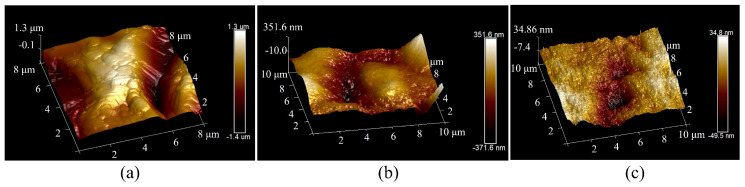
Three-dimensional surface morphology formed under different ultrasonic vibrations. (a) Original surface. (b) Ultrasonic longitudinal vibration. (c) Ultrasonic longitudinal–torsional vibration.
